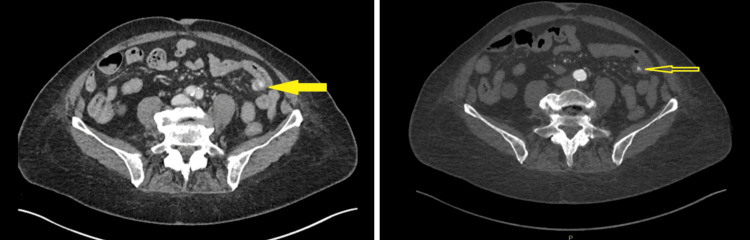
Sagittal CTA images showing active jejunal diverticular bleeding (yellow arrows) (A) Initial sagittal slice showing contrast extravasation in the jejunum. (B) Adjacent sagittal slice highlighting the same bleed at a slightly different level. CTA: computed tomography angiogram
